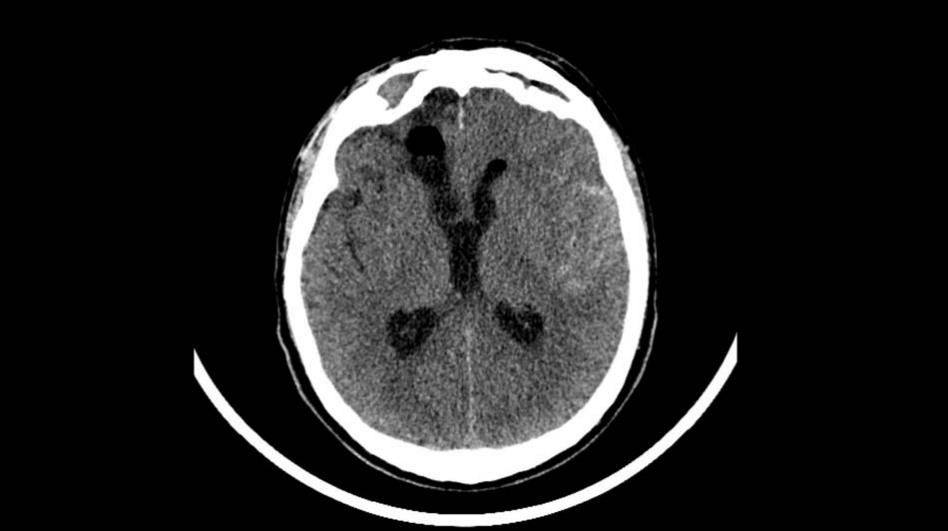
Head CT—state before VP shunt implantation. (Department of Neurosurgery own material).
